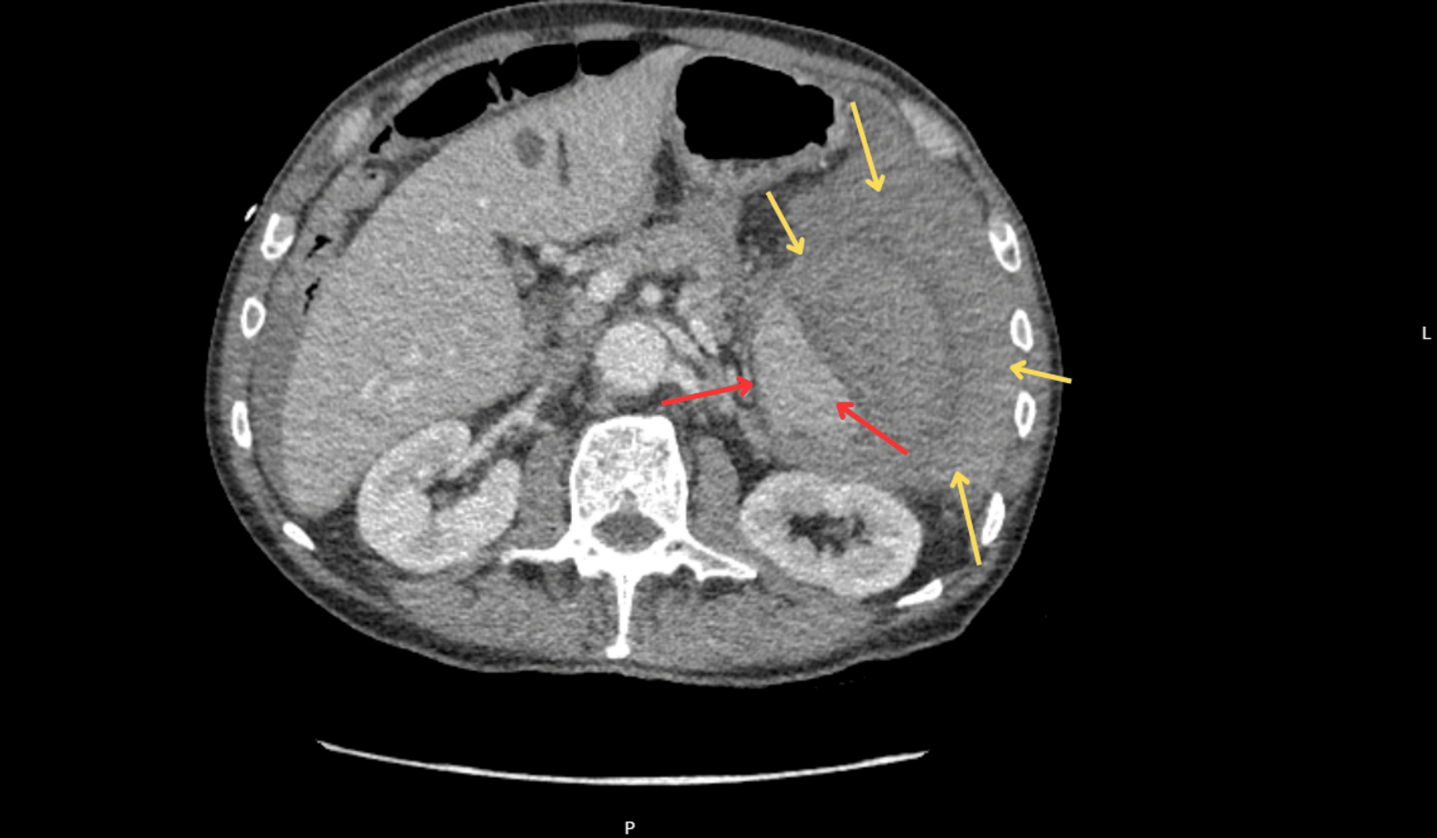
Contrast-enhanced CT of the abdomen showing the spleen (red arrow) and perisplenic fluid collection (yellow arrow), consistent with hemoperitoneum Axial CT image of the abdomen demonstrating a large perisplenic hematoma (yellow arrow) and free intra-abdominal fluid, consistent with hemoperitoneum. These findings are indicative of a spontaneous splenic rupture. CT: computed tomography
